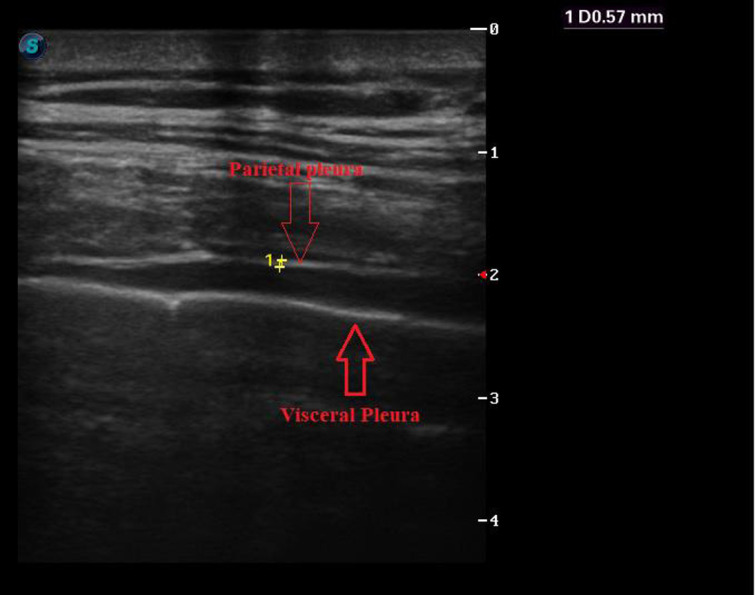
Ultrasonographic Imaging of the parietal and visceral pleura using a linear array transducer at 10 MHz on superficial mode (visceral and parietal pleura are indicated with red arrows).
